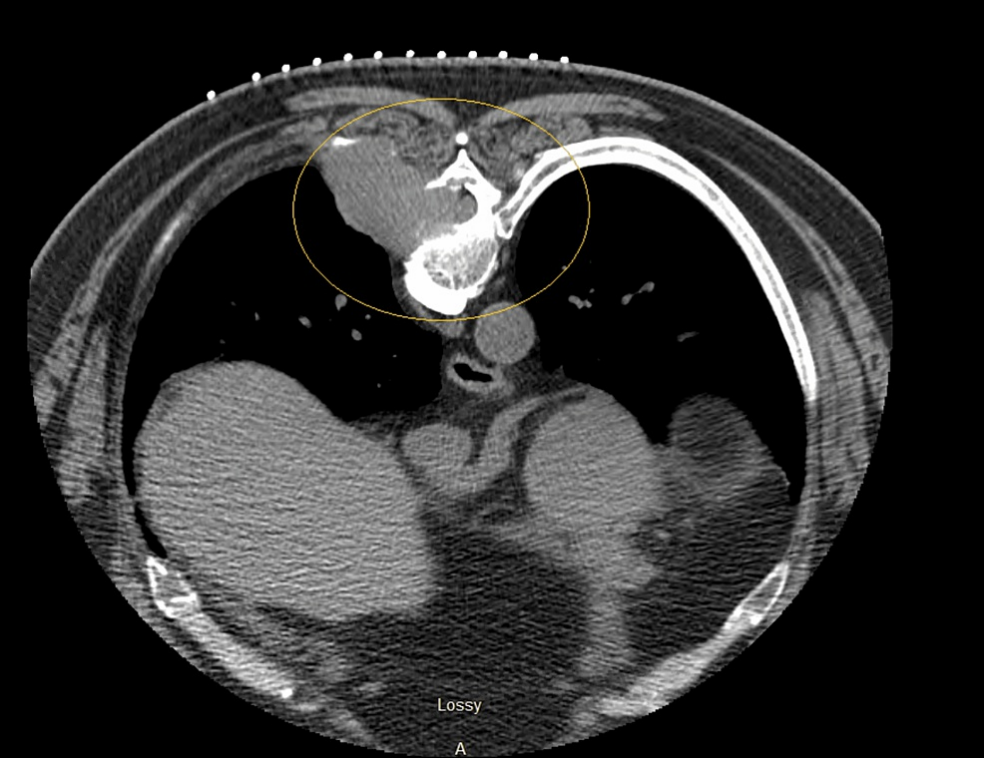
CT-guided core needle biopsy of the 5.6×3.5×3.2 cm mass lesion of the T8 vertebral body. CT: computed tomography.
